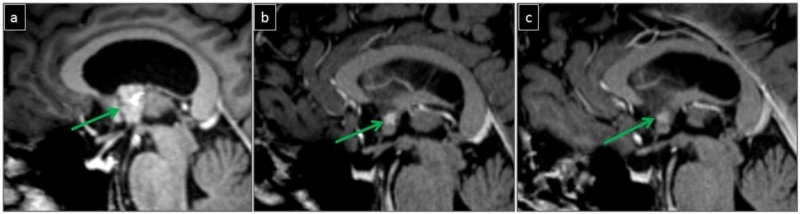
MRI brain (a-c). Sagittal T1-fat saturated post-contrast images from the preoperative scan showing the enhancing mass in the anterior third ventricle (green arrow in a). Initial post-operative scan after six weeks shows a tiny enhancing residual component (green arrow in b), which is stable in the follow-up scan after one year (green arrow in c)
